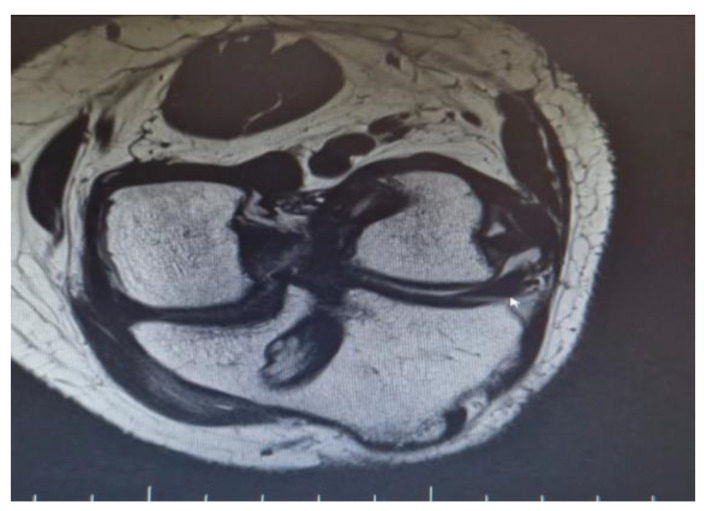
MRI demonstrating a single Achilles allograft used to reconstruct both the ACL and MCL, showing graft positioning and fixation.
